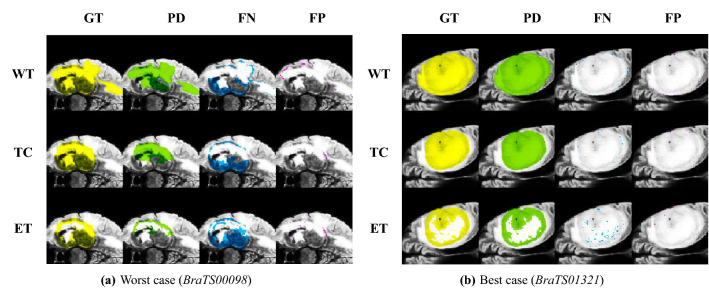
Segmentation of WT, TC and ET by Net1-Net3 of MRI images in FLAIR values for (a) the worst case (*BraTS00098*) and (b) the best case (*BraTS01321*).
